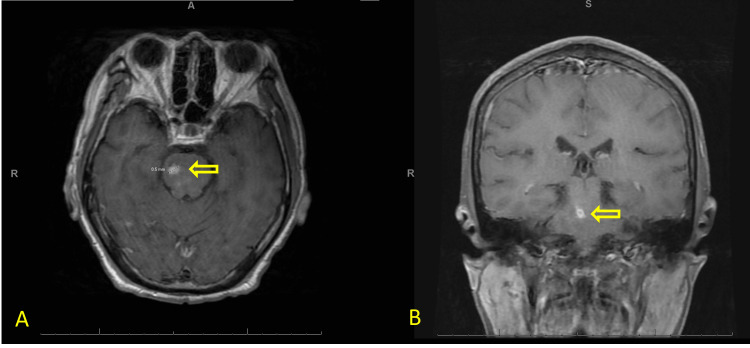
Postoperative head MRI with T2 fluid-attenuated inversion recovery sequence showing reduced ring-enhancing lesion in right pons (yellow arrow) in both axial (A) and coronal (B) views.
